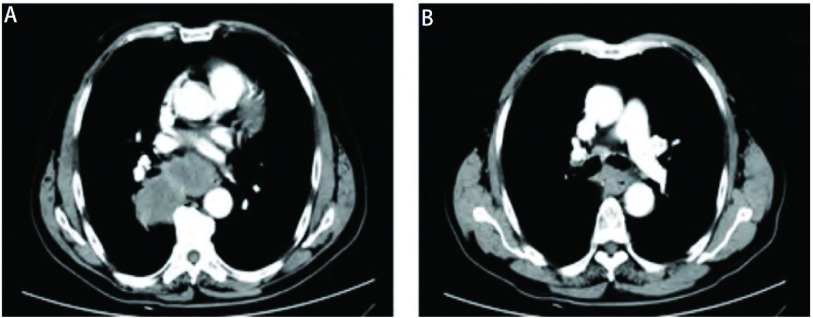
胸部CT。A：右肺门、纵隔淋巴结增大融合；B：食管中下段管壁增厚。 Computed tomography of the chest. A: The fused enlargement of lymph nodes in right hilar and mediastinum; B: The thickened wall of lower esophagus.
